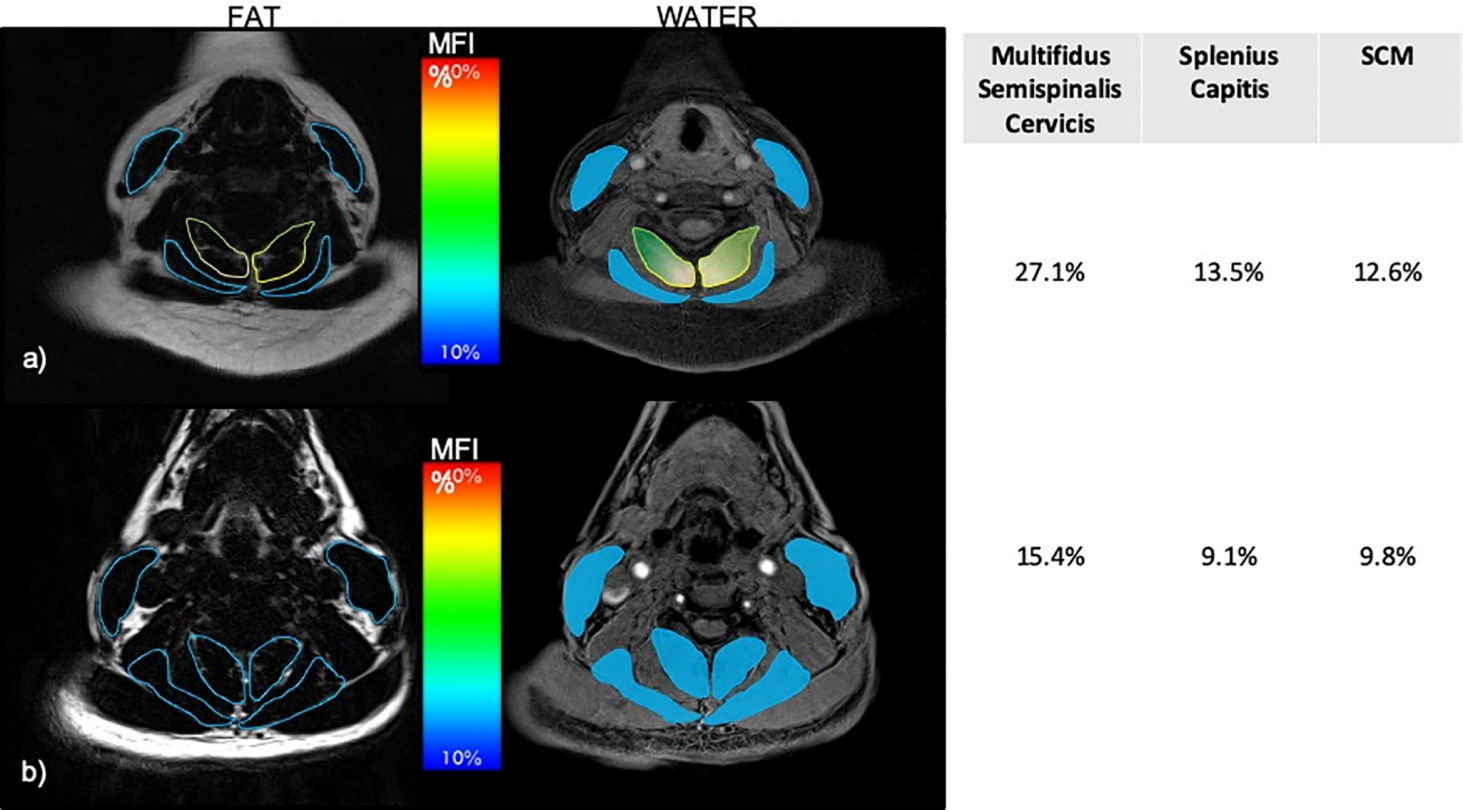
Fat/water MRI of the deep and superficial neck muscles at C5 within one-week of the MVC. a) represents the 32-year-old female with poor recovery at 12-months post-MVC with corresponding MFI values (%). b) represents the 50-year-old male reporting full-recovery at 12-months post-MVC with corresponding MFI values (%*)*.
